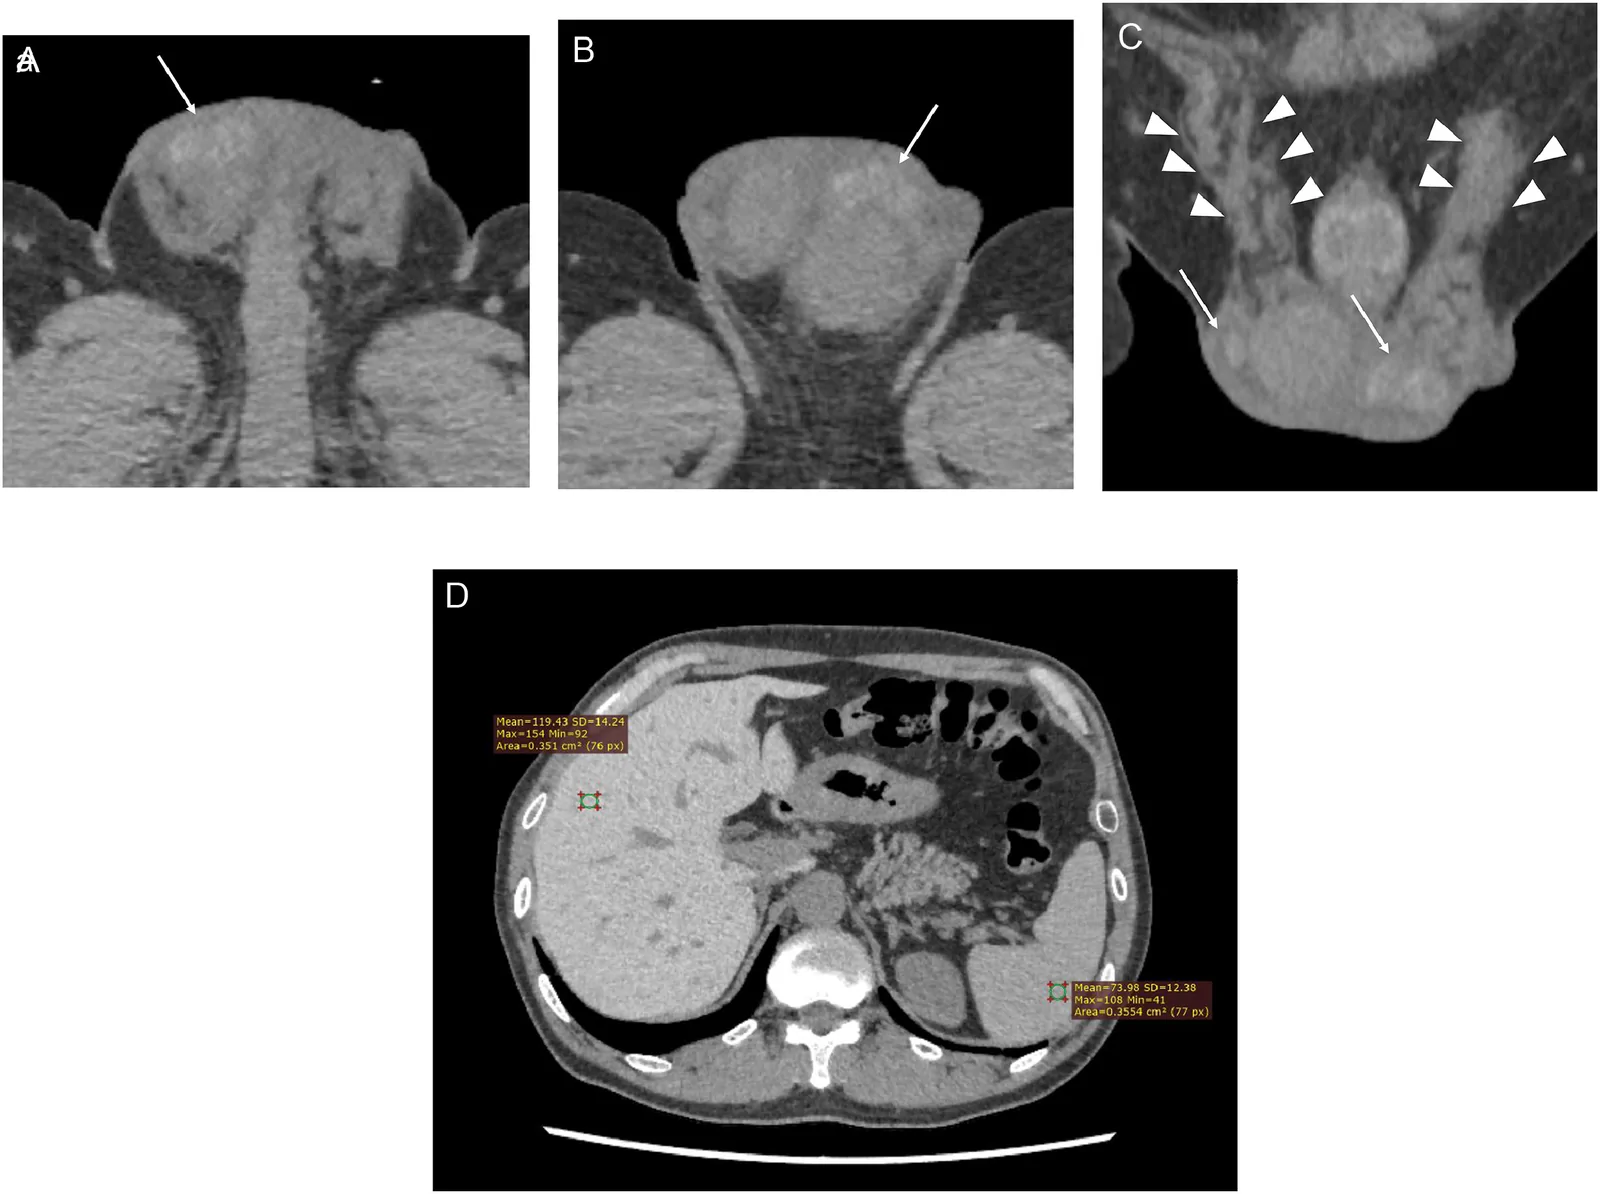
Noncontrast CT of the abdomen and pelvis. (A and B) Axial images show hyper attenuating right (A) and left (B) epididymides (arrows). (C) Coronal image shows hyper attenuation of the bilateral epididymides (arrows) and swelling of the bilateral spermatic cords (arrowheads). (D) Axial image at the level of the liver with regions of interest placed in the liver and spleen; hepatic attenuation (mean 119.4 HU) is increased relative to the spleen (74.0 HU), consistent with amiodarone deposition.
